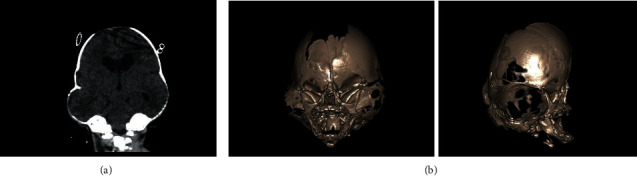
(a) CT scan of the head showing marked cloverleaf deformity with lateral ventricle dilatation. (b) Three-dimensional craniofacial reconstruct computed tomography of the skull (AP and lateral) demonstrating early closure and overlapping of all the cranial sutures with cloverleaf deformation.
